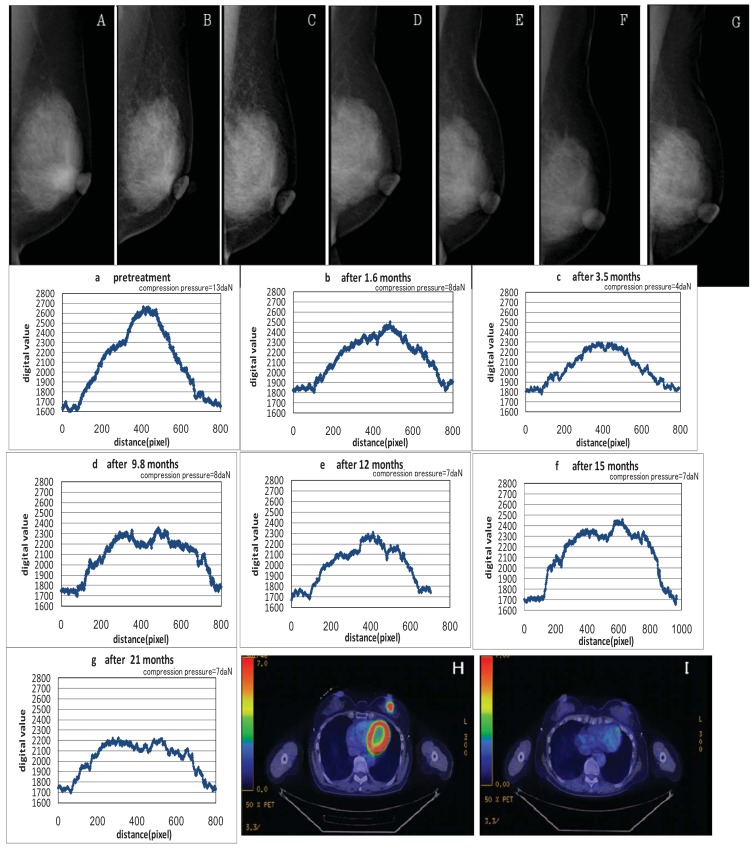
Case 4. 59 years old female patient with left breast cancer (cT2N0M0) treated with radiosensitization (KORTUC II). A ∼ G: Pre and post-KORTUC II treatment evaluation of changes of tumor shadows on MMG. a ∼ g: Profile curve of changes of tumor shadows (1 pixel = 93.9 μm). H, I: PET-CT images before KORTUC II (H) and 28 months after KORTUC II (I). (H) Tumor diameter is 27 mm (SUV_MAX_ = 8.1). (I) There is neither local recurrence nor distant metastasis.
